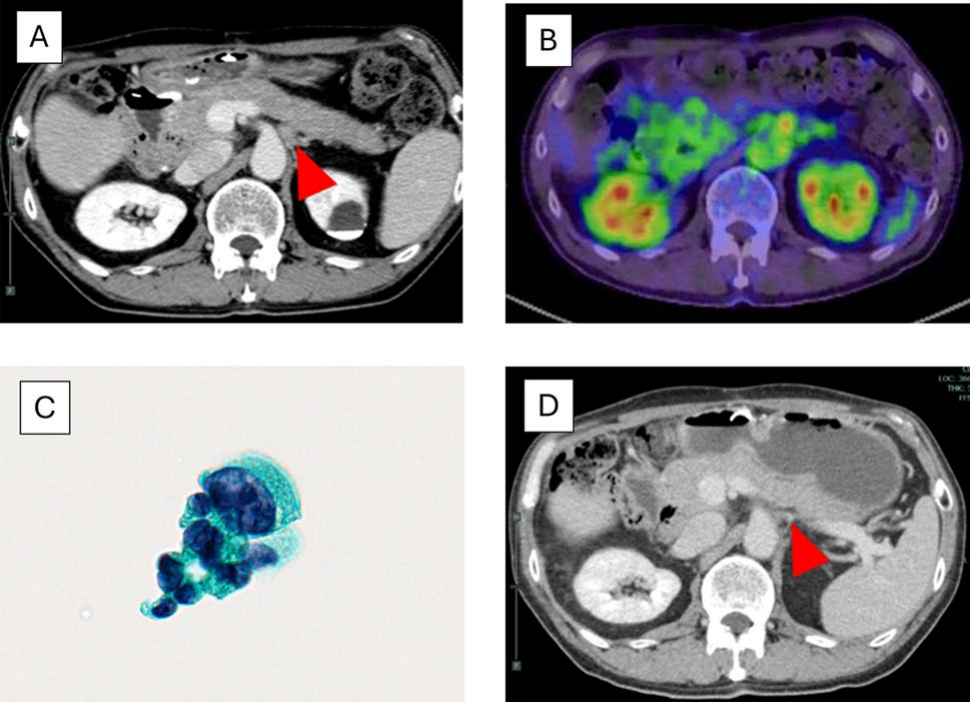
Imaging series after tumor recurrence. A At 20 months after surgery, contrast-enhanced abdominal CT (axial view) revealed increased soft-tissue density around the aorta (arrowhead). B FDG-PET showed increased uptake in that region. C Endoscopic ultrasound-guided biopsy was performed at the same site, and the cytological examination revealed atypical cells, leading to a Class V diagnosis. D At 28 months postoperatively, contrast-enhanced CT showed shrinkage of the lesion (arrowhead)
